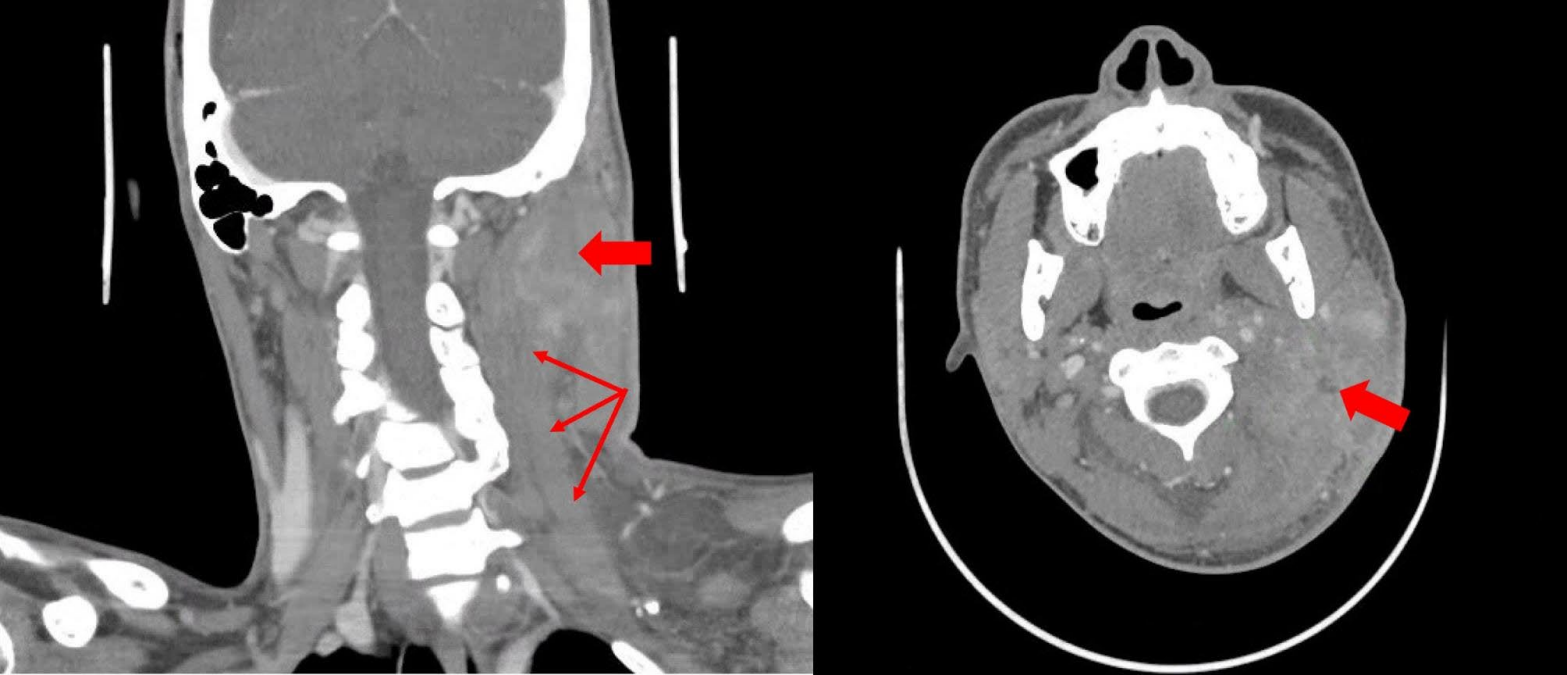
CT Head and Neck demonstrating enhancing soft tissue phlegmon with central necrosis (wide arrows) in the L posterior neck with superimposed myositis of the sternocleidomastoid and paraspinal muscles and soft tissue inflammation, as well as multiple enlarged cervical lymph nodes (thin arrows)
